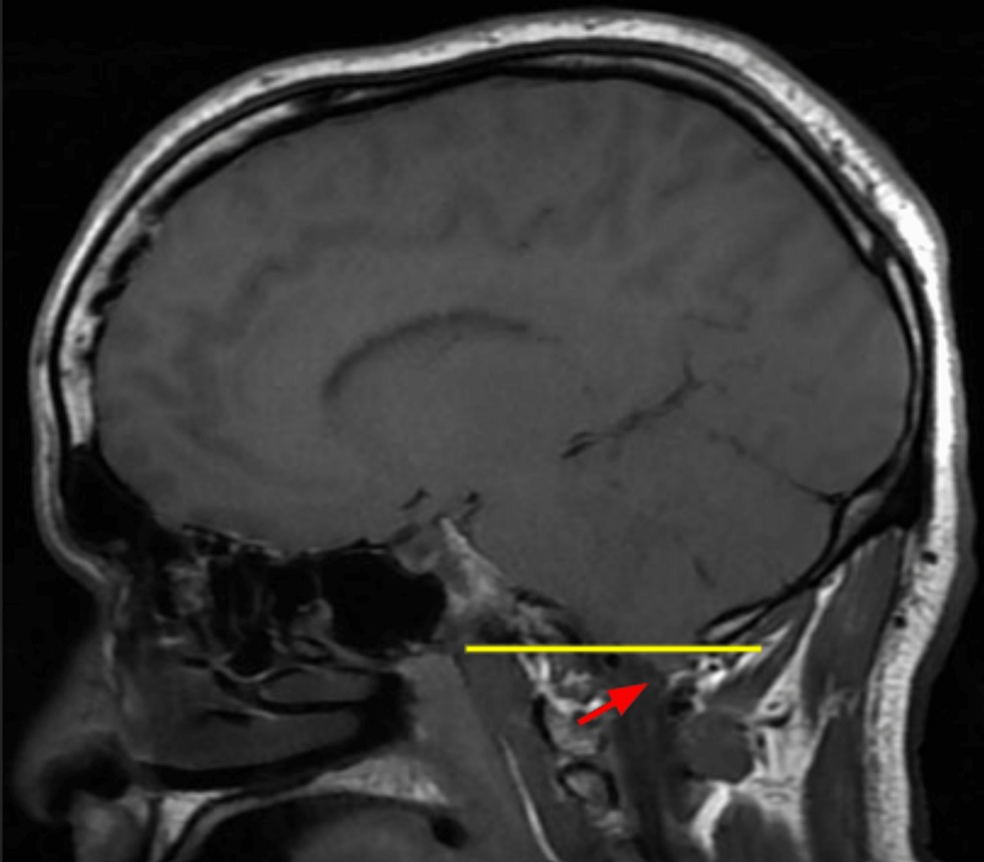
MRI brain T1 weighted series showing cerebellar tonsils herniated below foramen magnum
